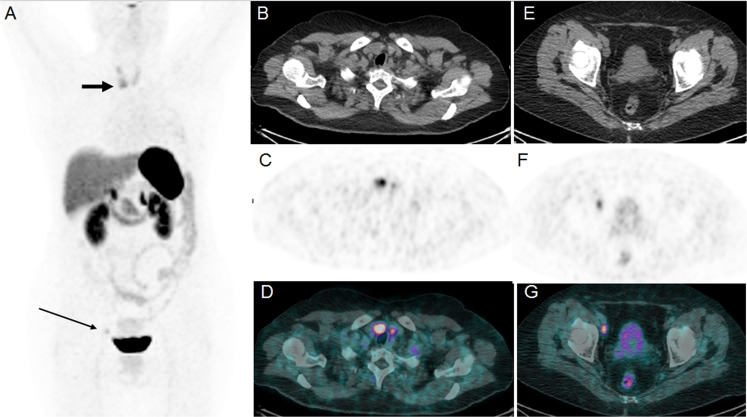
Maximum intensity projection (MIP) showing a mild increased DOTATOC uptake on right iliac lymph node (slim arrow) and focal high uptake on the right thyroid lobe (thick arrow) (A). Axial noncontrast enhanced CT showing a nodule of 18 mm on the lower part of the right thyroid lobe (B), axial PET (C) and PET/CT fused images with the increased uptake corresponding to the nodule detected by CT (D). Axial CT (E), PET (F) and PET/CT fused (G) images showing a right external iliac lymph node
